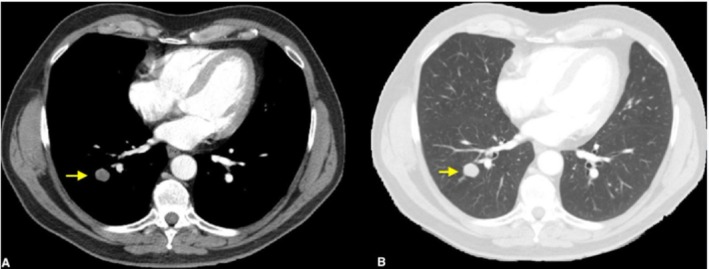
Chest CT scan showing a dense nodule of 18 × 15 mm of the right lower pulmonary lobe. (A) Mediastinal window; (B) parenchymal window.
